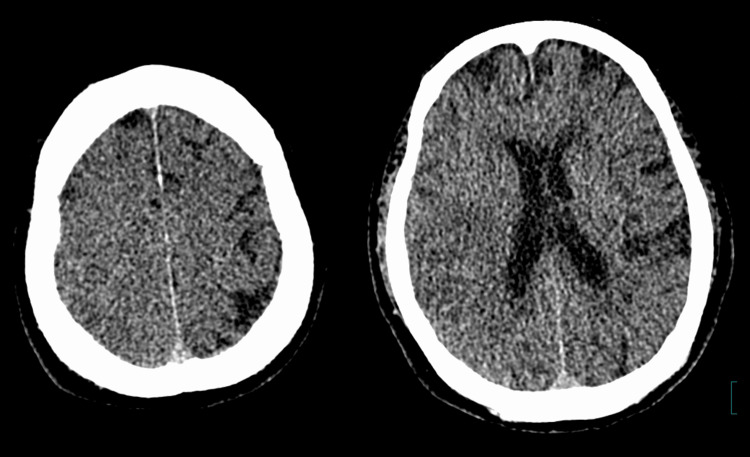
Virtual non-contrast images taken using dual-energy CT In virtual non-contrast images taken using dual-energy CT, the high-density areas seen on plain CT were iso-dense.
